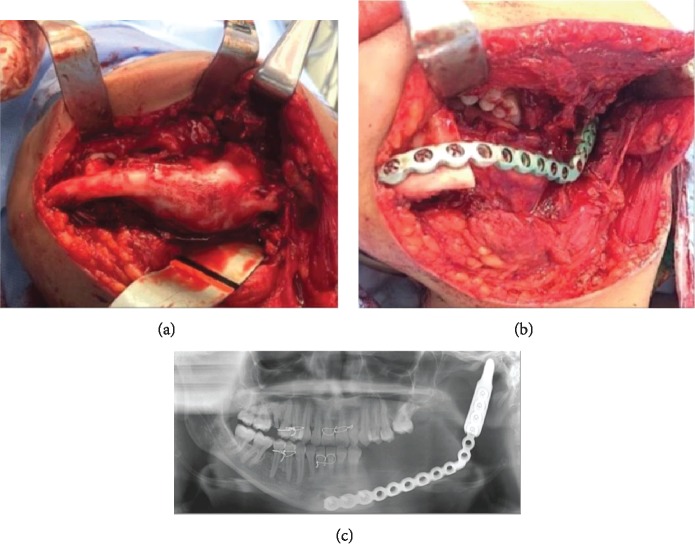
(a) Photograph showing the tumor and the expansion of both the buccal and lingual cortices. (b) Segmental resection of the left mandible stabilized with a 2.7 mm reconstruction plate. (c) Postoperative orthopantomogram.
